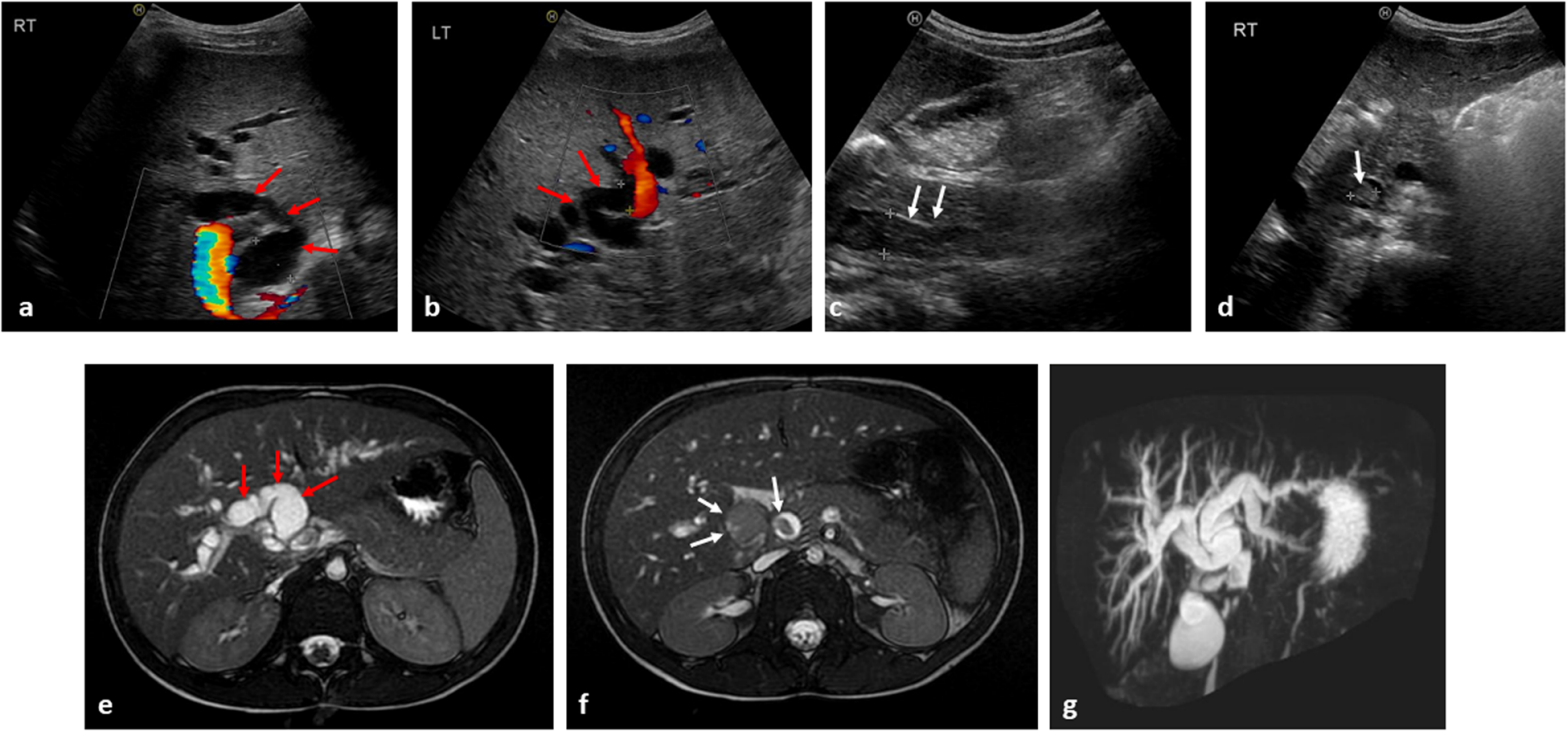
A 6-year-old girl, also presenting with jaundice from a biliary rhabdomyosarcoma. a, b Transverse ultrasound images at diagnosis reveal marked intrahepatic biliary ductal dilatation (red arrows) measuring > 1 cm in diameter within both the right and left hepatic lobes. c, d Upon careful assessment of the common bile duct to its origin, a homogenous intra-ductal mass is identified (white arrows). e, f Axial T2-weighted MRI images through the liver, subsequently confirm the intrahepatic ductal dilatation (red arrows), with intra-ductal mass (white arrows) in keeping with the biliary rhabdomyosarcoma. g The 3D-MIP from the MRCP again helps to demonstrate the extent of the intrahepatic biliary ductal dilatation. In this clinical scenario, compared to the images of Fig. 15, the intra-ductal mass is harder to visualise on ultrasound without careful assessment of the common bile duct to its origin, highlighting the importance of careful assessment and patience to ensure all pertinent findings are identified
